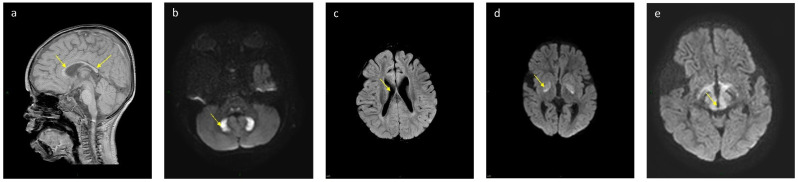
Representative Brain MRIs: (a) partial agenesis of corpus callosum. (b) DWI restriction of dentate nucleus. (c) moderate ventriculomegaly. (d) 2-DWI restriction of globus pallidum. (e) 2-DWI restriction of midbrain.
